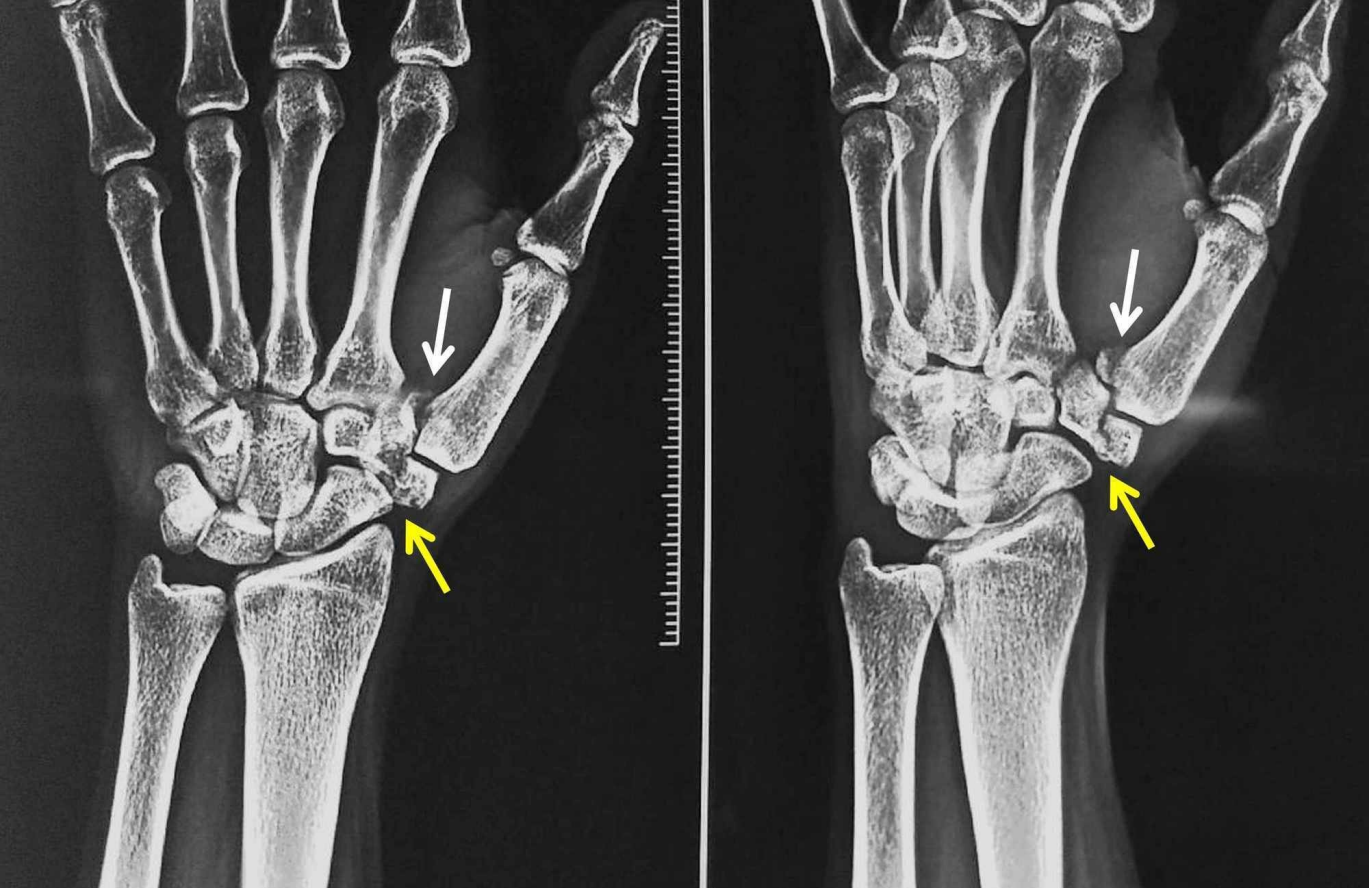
Anteroposterior and oblique radiographs of the wrist and hand White arrows - Bennett fracture; yellow arrows - trapezium fracture
